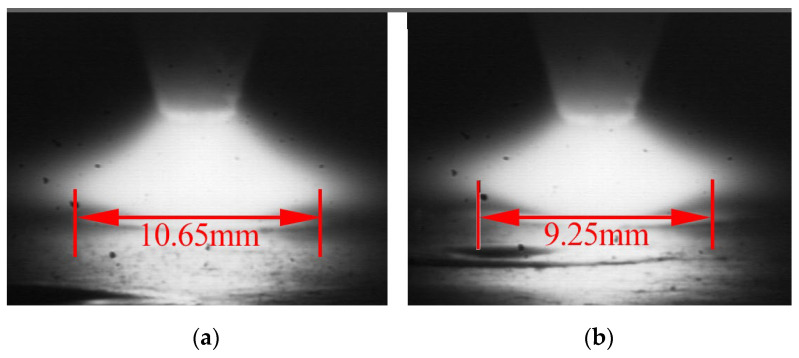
Arc morphology under different ultrasonic frequency pulse current: (a) conventional TIG; (b) ultrasonic frequency pulse current 30 A.
